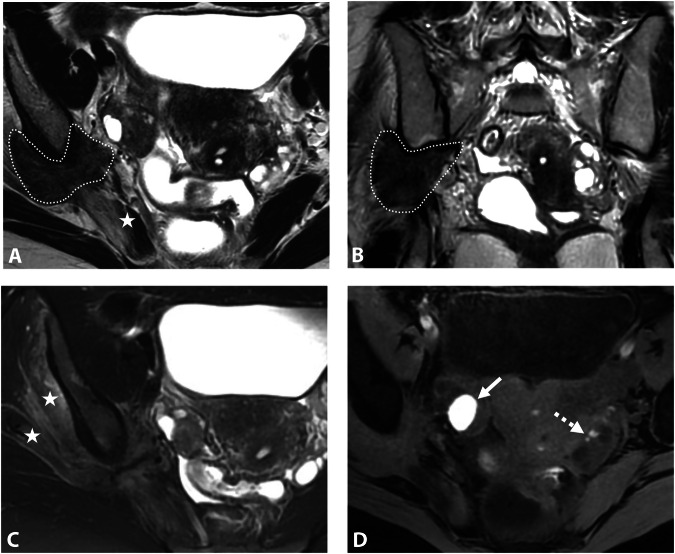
DE in a 31-year-old woman with right sciatica initially cyclical, then became chronic with walking difficulties. A Axial and (B) coronal T2W MR images show an infiltrative endometriotic mass of 5 cm (measure not shown) (dotted line) centered on the right sciatic notch with involvement of the sciatic nerve. Note the hypertrophy of the piriformis muscle (A, star). C Axial fat-suppressed T2W MR image shows denervation of the gluteus medius and minimus muscles (stars), indicating the involvement of the superior gluteal nerve in the sciatic notch, as well as the piriformis muscle. D Axial fat-suppressed T1W MR image shows a right-sided endometrioma (arrow) and hemorrhagic implants of the left ovary (dashed arrow). Surgical intervention was not possible due to the intrinsic involvement of the sciatic nerve, which could risk nerve damage. The patient was treated medically with an LHRH analog
